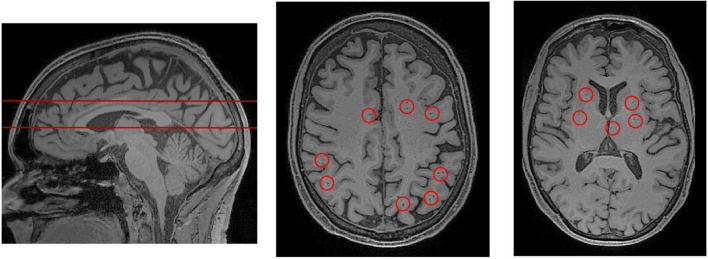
PVS rating scales rely on representative axial slices to assess the severity of PVS enlargement. (Left) A sagittal view of the brain, from a T1-weighted MRI scan. The red lines indicate the axial slices that have been selected for visual PVS rating. The upper line was chosen to assess PVS in the centrum semiovale, and the lower line was chosen for the basal ganglia. The centrum semiovale is the mass of white matter above the lateral ventricles. (Middle) An axial slice of the centrum semiovale corresponding to the top line. (Right) An axial slice of the basal ganglia corresponding to the bottom line. Visible perivascular spaces are outlined by red circles. For an extensive review of rating scales, please refer to Paradise et al. (2020).
